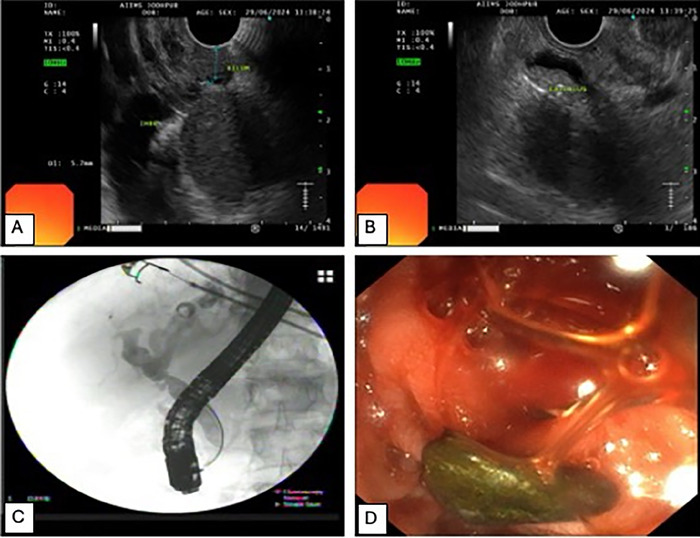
(A, B) Endoscopic ultrasound images show eccentric thickening of the proximal common hepatic duct with upstream ductal dilatation and distal common bile duct calculi. (C) Endoscopic retrograde cholangiopancreatography image shows a dilated common bile duct with intraluminal filling defects consistent with choledocholithiasis. (D) Endoscopic view shows extraction of a bile duct calculus during endoscopic retrograde cholangiopancreatography.
